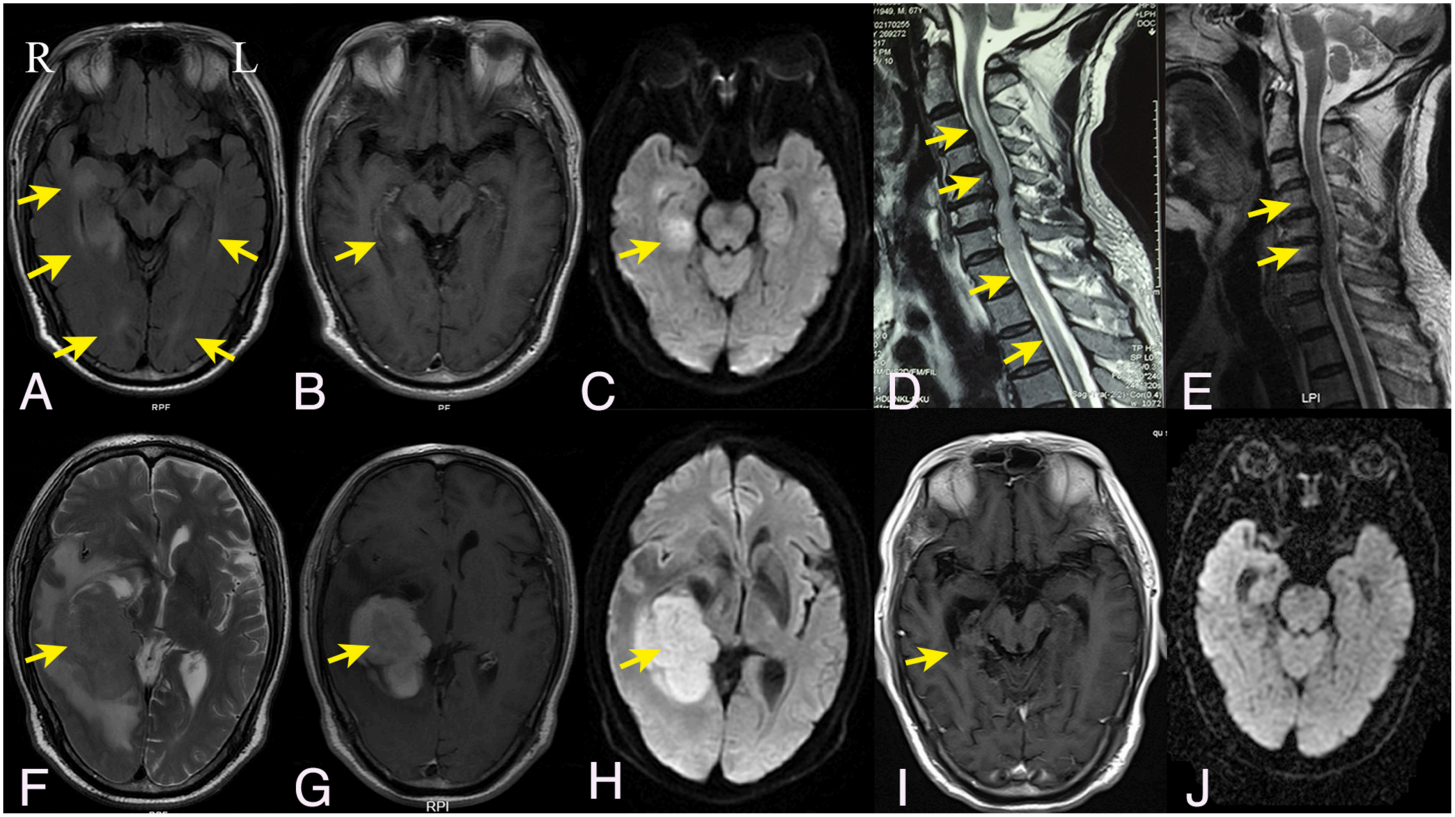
Brain and spine MRI findings. The initial axial fluid-attenuated inversion recovery (A), axial T1-weighted gadolinium-enhanced (B), and axial diffusion weighted imaging (C) brain MRI showed bilateral irregular hyperintense lesions around the ventricles with enhancement on the right temporal lobe. (D) Sagittal T2-weighted cervical spine MRI showed hyperintensity longitudinally extending from the cervical to the thoracic cord. (F–H) Six months later, the follow-up axial T2-weighted brain MRI (F) and diffusion weighed imaging (H) showed a mass lesion in the right temporal lobe with homogenous enhancement on T1-weighted gadolinium-enhanced series (G); Sagittal T2-weighted cervical spine MRI showed regredient lesions in the cervical cord (E). (I,J) After the chemotherapy, the right temporal lesion on T1-weighted gadolinium-enhanced series (I) was significantly regredient and necrotic; No abnormal signal was displayed on diffusion weighted imaging (J). R indicates right and L indicates left.
